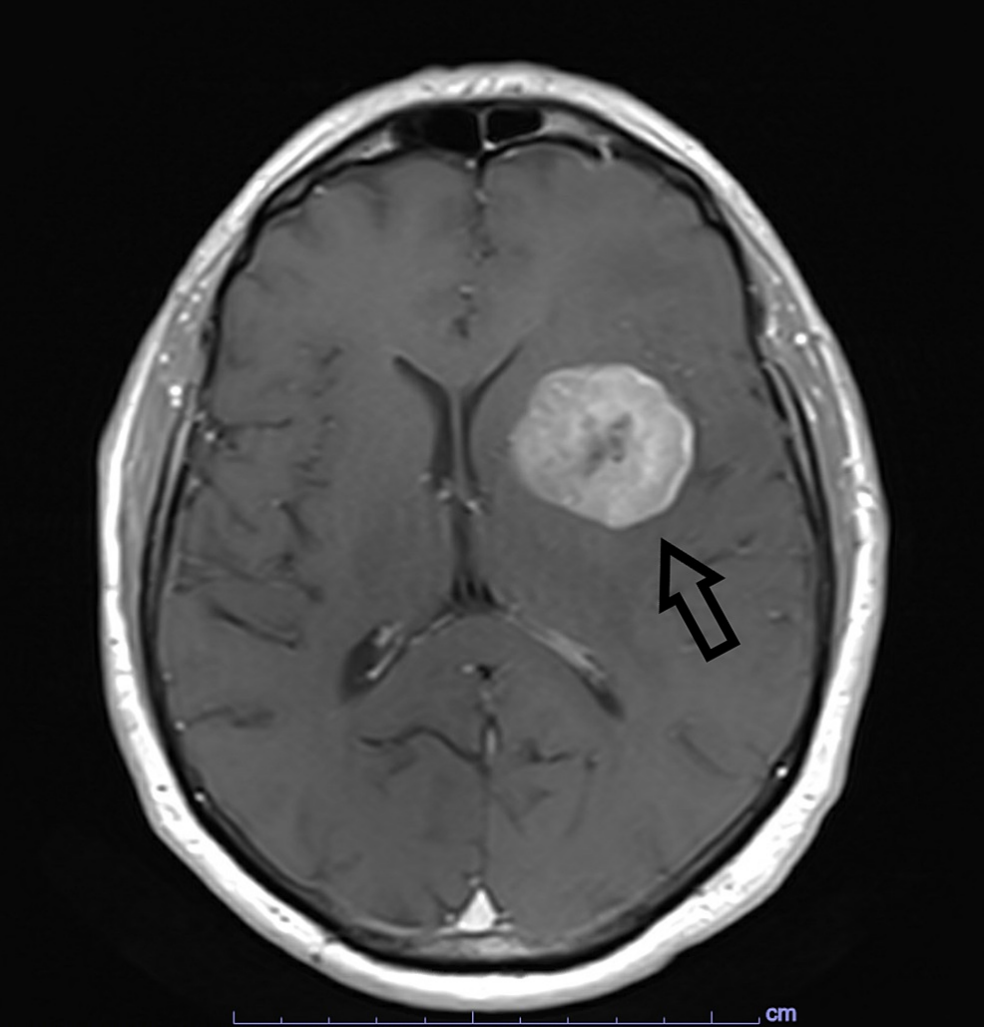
Pre-operative, T1-weighted, axial MRI image with contrast demonstrating left frontal lesion. Arrow: left frontal lesion
